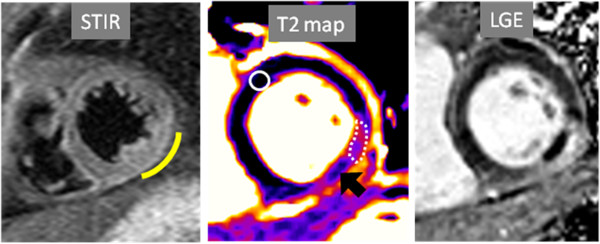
T2-weighted short tau inversion recovery (STIR), T2 map and late gadolinium enhancement (LGE) images – all obtained in a similar mid-short axis plane – are shown from CMR examination performed in a 53 year-old male who suffered ST-elevation myocardial infarction. CMR performed 1 day following percutaneous coronary revascularization of a large obtuse marginal coronary artery yielded a calculated LVEF of 55%. STIR imaging suggested increased signal intensity in the inferolateral wall (arc), but does not demonstrate an abnormality within this region. Quantitative T2 mapping shows a region of increased T2 with a hypointense core indicating microvascular obstruction (MO). Mean T2 values in the edematous region (dotted line), MO (black arrow) and remote myocardium (solid circle) were 71, 59 and 48 msecs. 10 min after intravenous gadolinium-based contrast administration, LGE confirms a region of MO in this post-STEMI patient. (Image provided courtesy of Subha V. Raman, MD, MSEE; Davis Heart and Lung Research Institute, The Ohio State University, Columbus, Ohio.).
